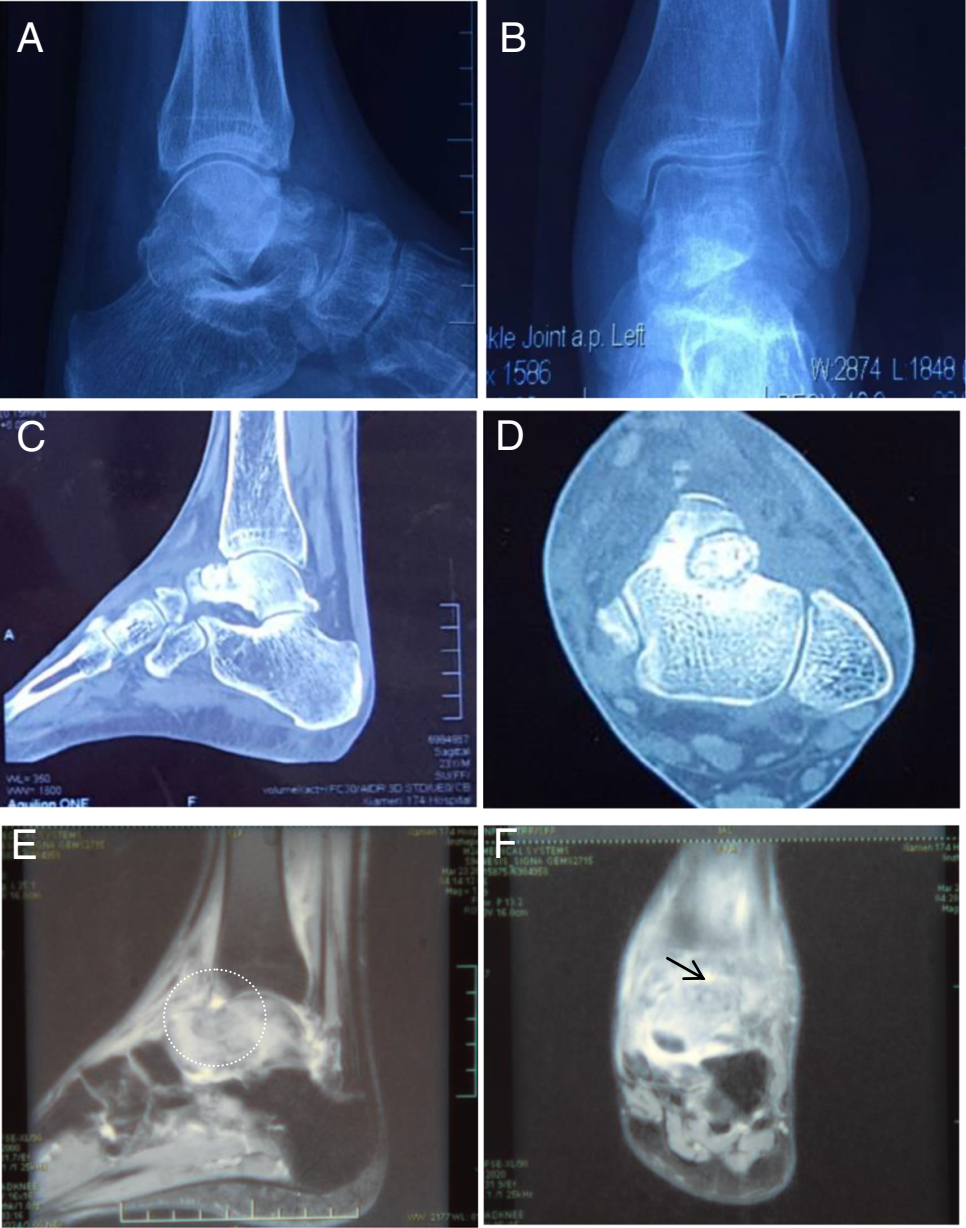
Preoperative imaging of the left ankle prior to the second surgery. (a, b) Preoperative radiographs show pathologic damage to the anterolateral talus, Approximately 1.0-cm-diameter higher-density nodules can be seen on the neck of the talus on the left side, with a clear boundary. (c, d) Preoperative computed tomography images show the characteristics of the osteoid osteoma nidus. (e, f) Preoperative sagittal T2-weighted and coronal T2-weighted magnetic resonance images show widespread bone marrow oedema of the talus with the osteoid osteoma lesion inside (white circle and black arrow indicate the nidus)
